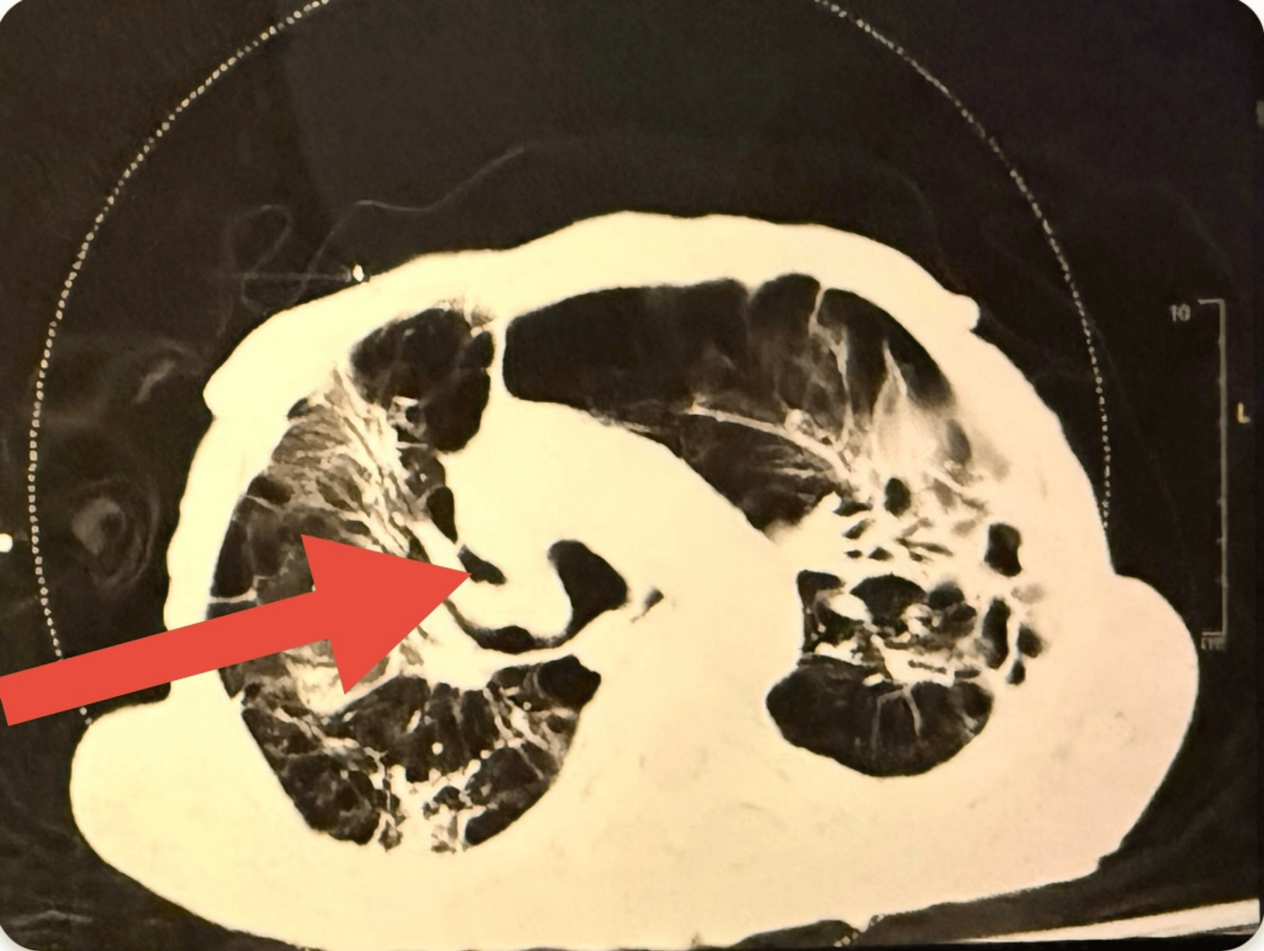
CT of the abdomen showing a 7.6 × 6.2 cm infrarenal abdominal aortic aneurysm. The red arrow indicates the abdominal aortic aneurysm.
